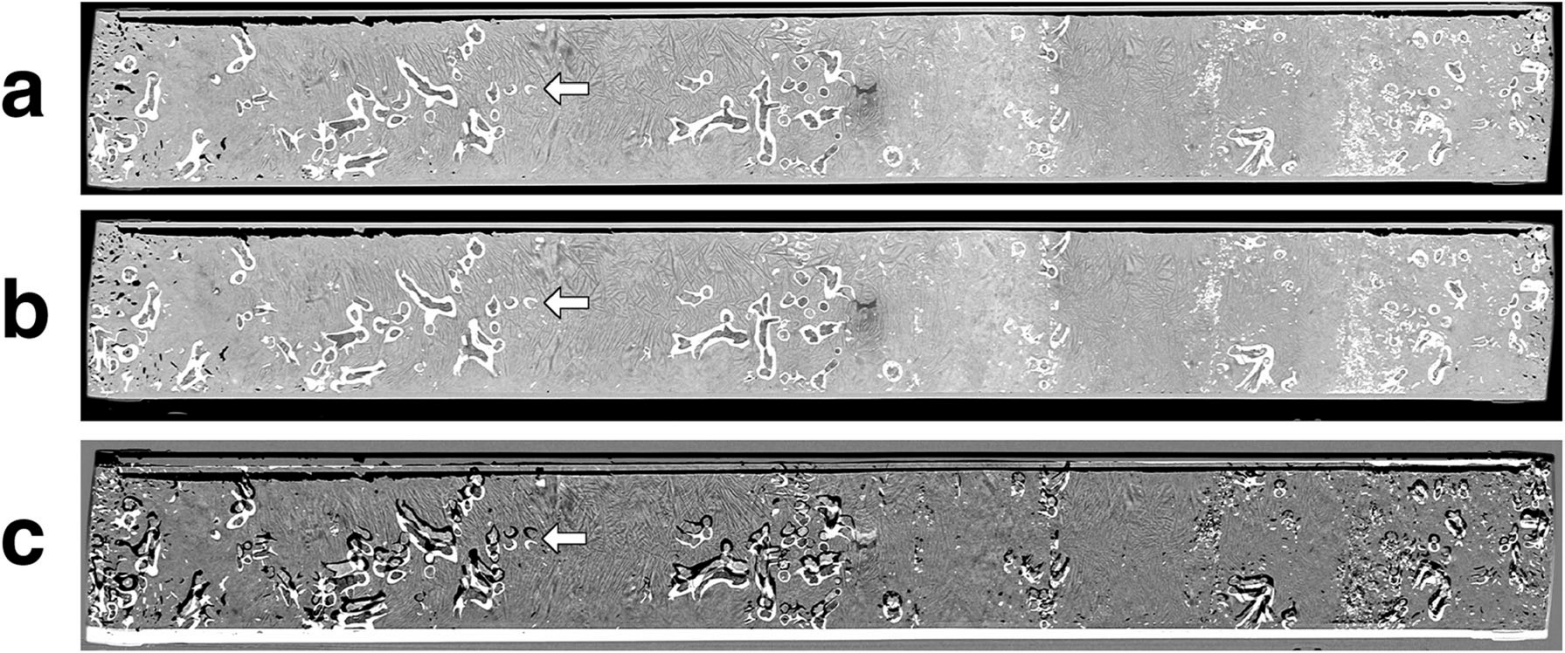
Examples of sagittal reconstructions of CT acquisitions of sediment cores, which were annotated with a digital ruler (not shown) and used during geological sampling to identify and locate coral fragments below the surface. a Before alignment correction. b After alignment correction. c Difference image before/after alignment correction. Areas with high density before correction and low density after correction are shown in white, areas with low density before correction and high density after correction are shown in black. Note the large differences in sample position in-plane and the smaller differences out-of-plane. For the effect of alignment correction on the sampling process, consider the coral fragment indicated by the arrow. As can be observed from the difference image, trying to sample this fragment at the location indicated by the uncorrected image would completely miss the fragment
